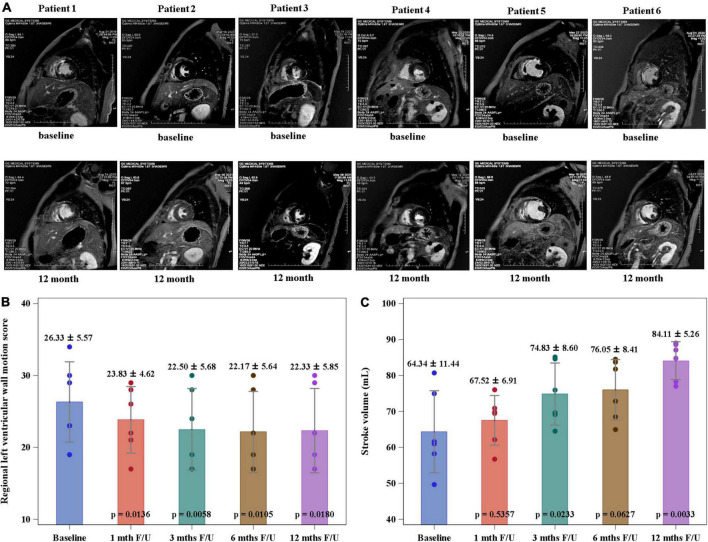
Regional left ventricular wall motion score (RLVWMS) and stroke volume evaluated by cardiac magnetic resonance imaging (CMRI). (A) The representative CMRIs of individual study patient at baseline and 12-month follow-up. The RLVWMS decreased (B) and the stroke volume increased (C) at 12-month follow-up compared to the baseline levels.
